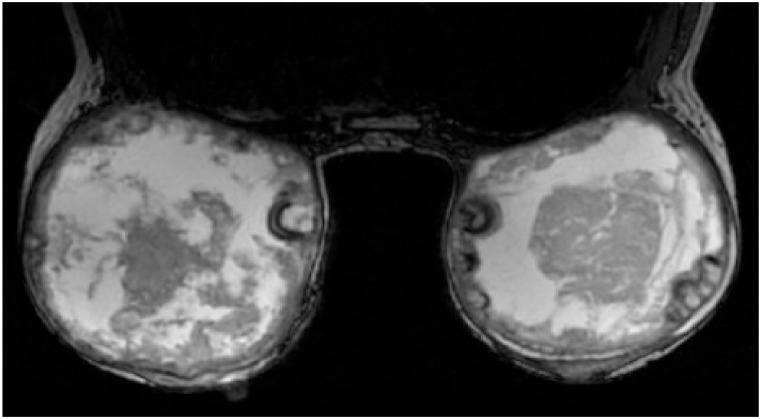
MRI of the patient’s breasts. On In-Phase axial T2 FSE-IDEAL ASSET imaging, the lesion demonstrated a mixed signal pattern with slightly hyperintense and hypointense components, with indistinct margins. Contrast-enhanced scanning revealed marked enhancement, and the time-signal intensity curve exhibited a plateau pattern.
